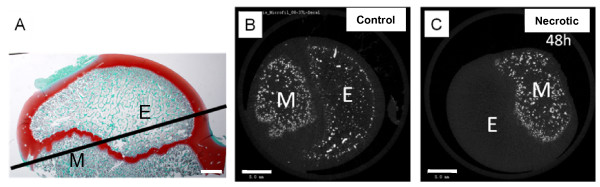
Optical and CT images of the proximal femur. The labels are E: epiphysis, and M: metaphysis. Bar = 5 mm. (A) Optical sagittal section with the transverse plane including the epiphysis and the metaphysis. (B) CT image of the control femoral head. (C) CT image of the necrotic femoral head.
